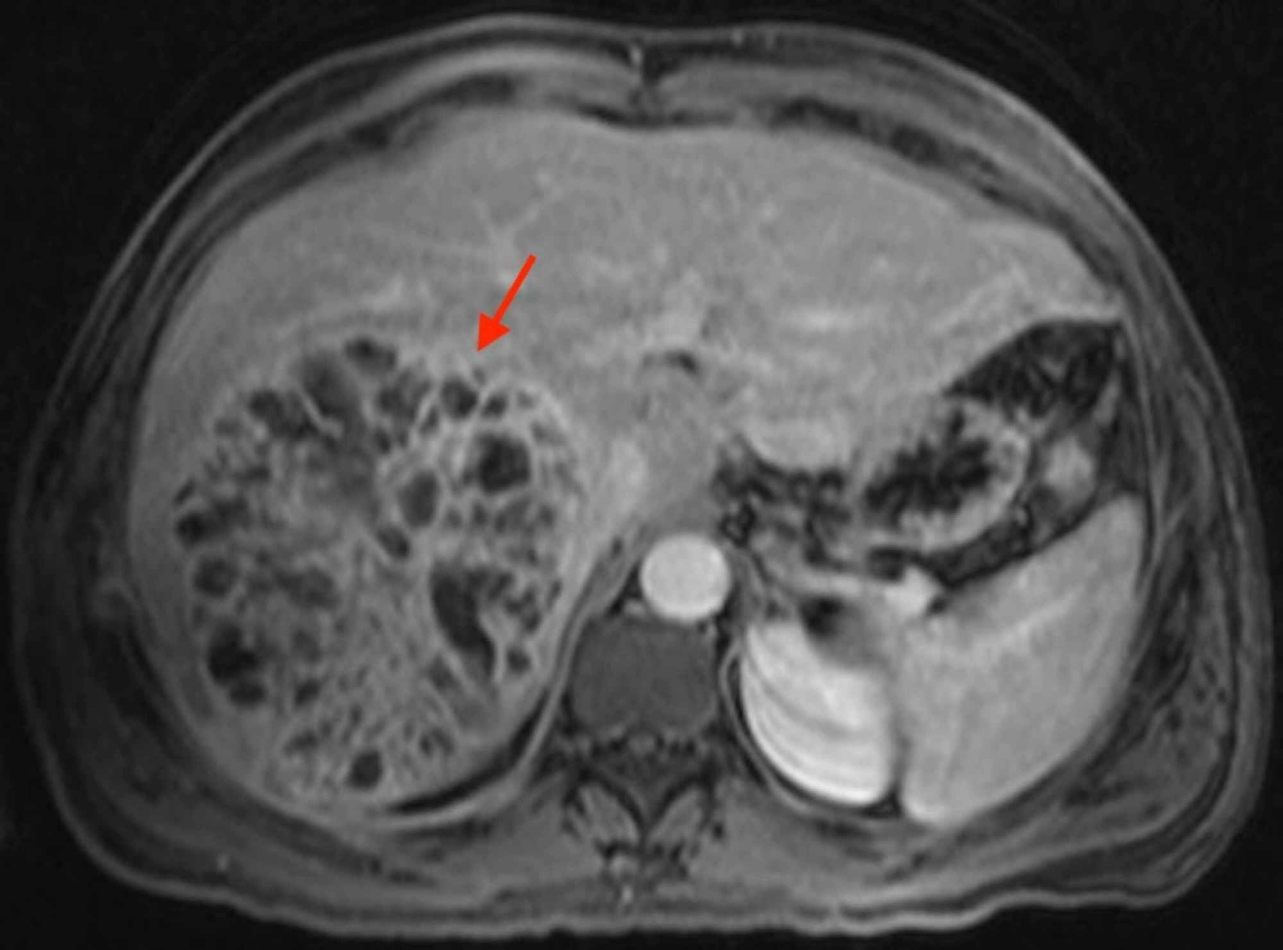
MRI of the abdomen demonstrating a 14.0 x 13.5 x 1.5 cm multicystic enhancing mass lesion within the segments 7 and 8 of the liver with an enhancing pseudocapsule. The arrow points to area of interest.
